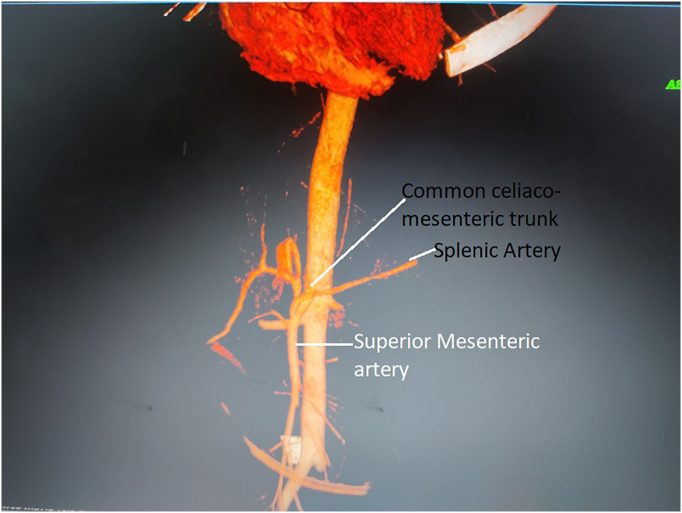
3-D reconstructed image of the CT angiography showing common origin of the CA and SMA, origin of the SA from the SMA, narrow angle between the CMT and abdominal aorta.
